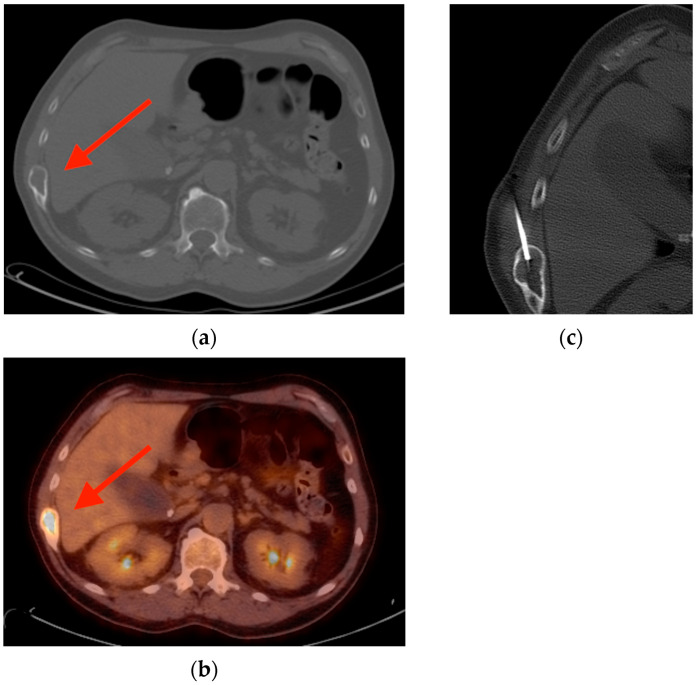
(a) CT scan of a 61-year-old patient with head and neck squamous cell carcinoma reveals an expansile lesion in the right rib (red arrow) with non-aggressive features including a well-defined sclerotic border and no periosteal reaction. (b) Corresponding FDG PET/CT imaging shows focal uptake in this same lesion (red arrow). (c) Despite its features, this lesion was later biopsied and found to be fibrous dysplasia. CT = computed tomography. FDG PET/CT = fluorine-18 fluorodeoxyglucose positron emission tomography/computed tomography.
